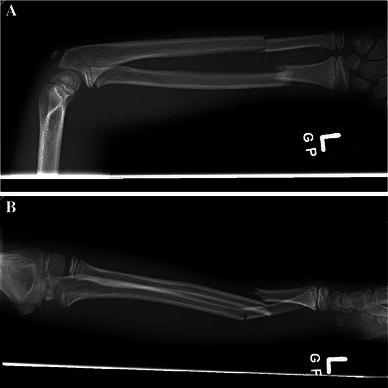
a, b Injury radiographs demonstrating displaced radius and ulna fractures
